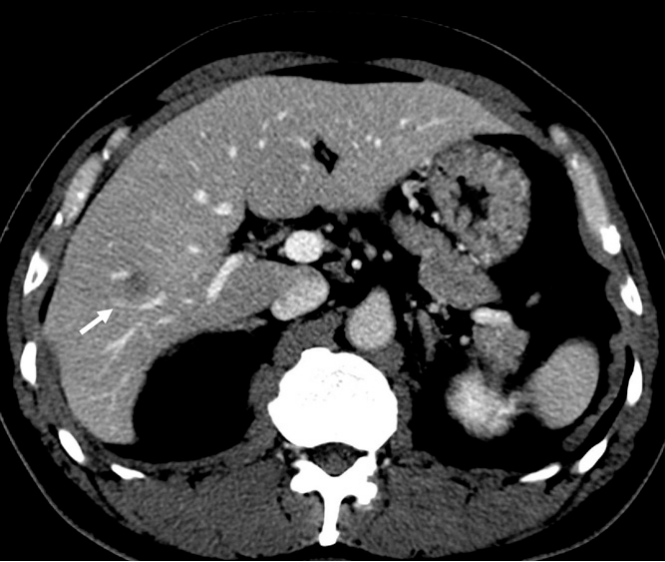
The CT image obtained 3 months later shows that the nodule migrates slowly upward and anteriorly in the liver (arrows).
